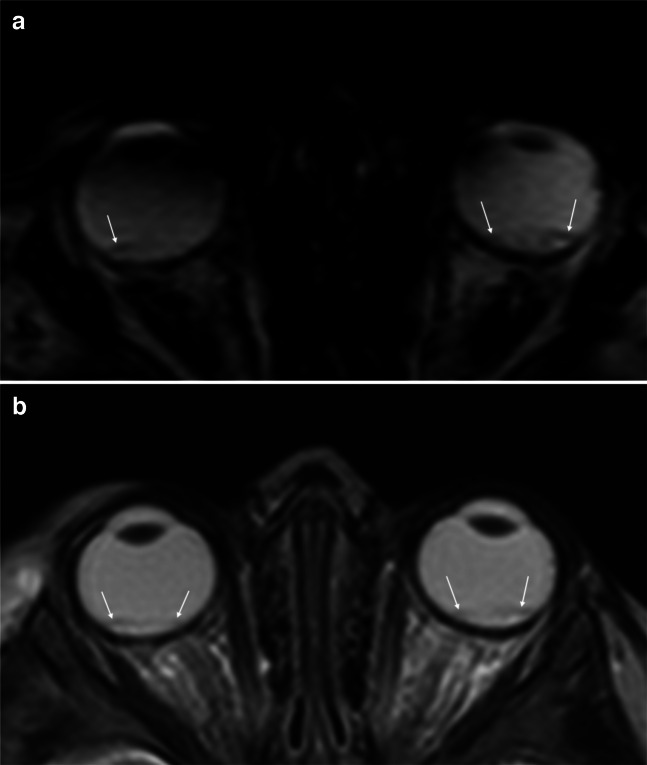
Six-month-old boy with abusive head trauma following shaking and left-sided retinal hemorrhages detected on fundoscopy. Ophthalmological assessment revealed localized hemorrhages in the left eye, with no hemorrhage detected in the right retina. a T2*-weighted magnetic resonance image and b T2-weighted magnetic resonance image show a distinct laminar elevation at the posterior pole of the retina in both eyes, highly consistent with retinal hemorrhages (arrows)
